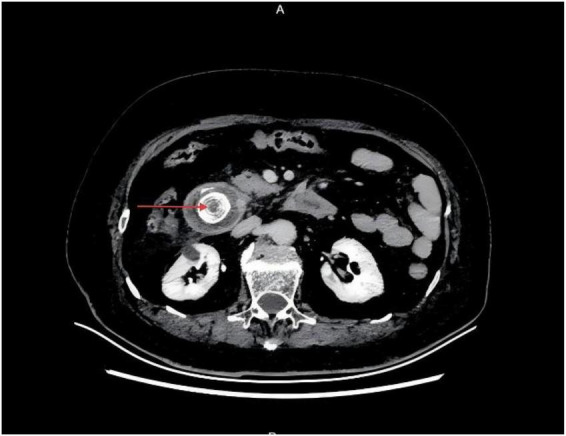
Axial abdominal computed tomography (CT) showing a ring-shaped hyperdense lesion within the duodenal lumen, indicating complete obstruction. Arrow indicates giant gallstone.
